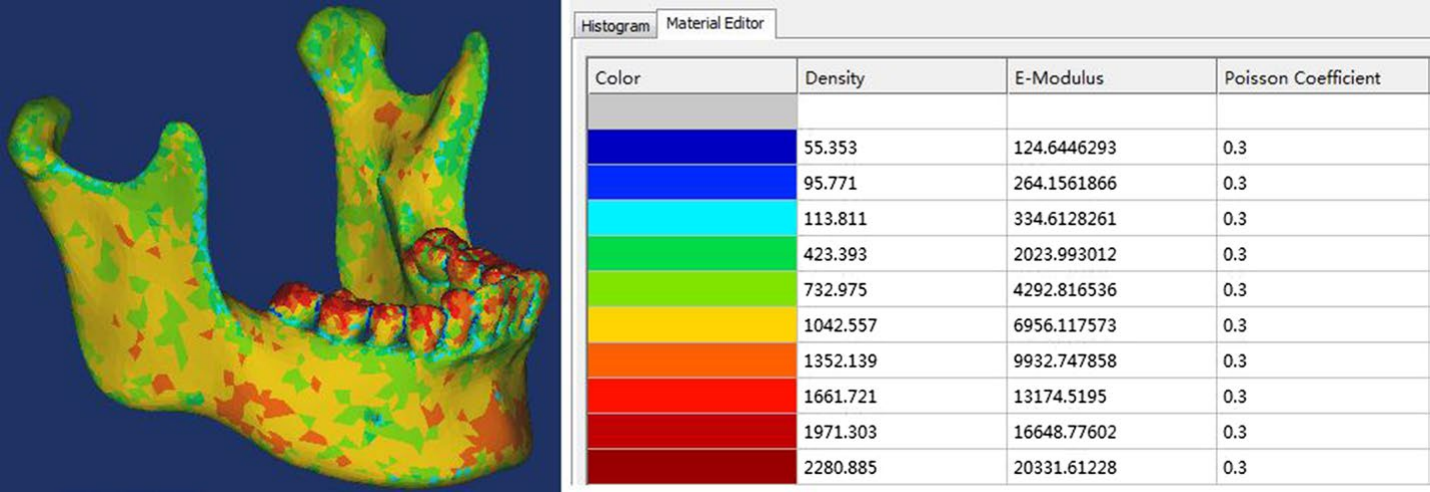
Material properties of the mandible model based on HU values from the CT images
